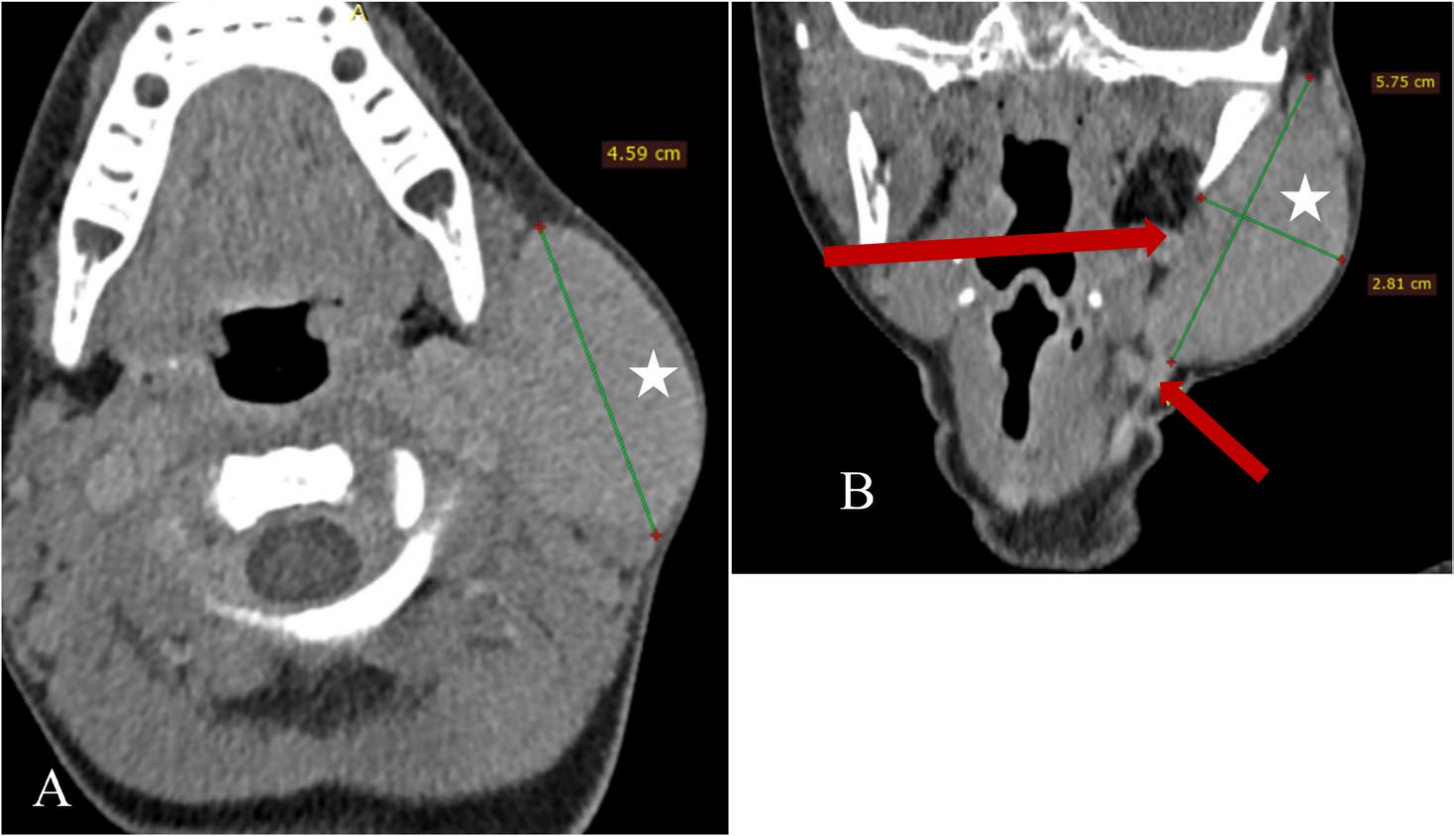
(A) and (B) are axial noncontrast CT of head and neck with a coronal reformat, respectively, showing an expansile soft tissue density well‐marginated mass (white star) in the left parotid region, which is nondestructive to the adjacent bone and does not breach the skin. It measures 5.75 × 4.59 × 2.81 cm. Red arrows show blood vessels.
